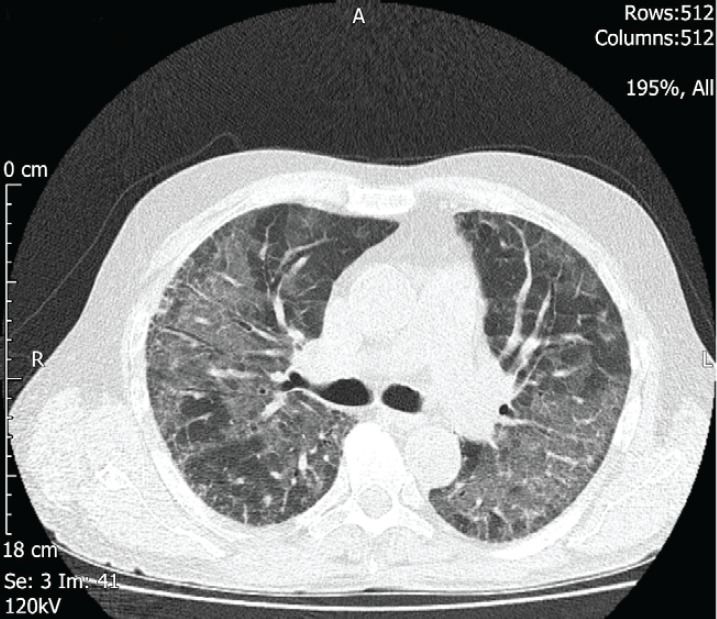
Panitumumab-induced fatal ILD. HRCT of the chest, axial image, two days after the admission, showing bilateral patchy ground glass changes.
